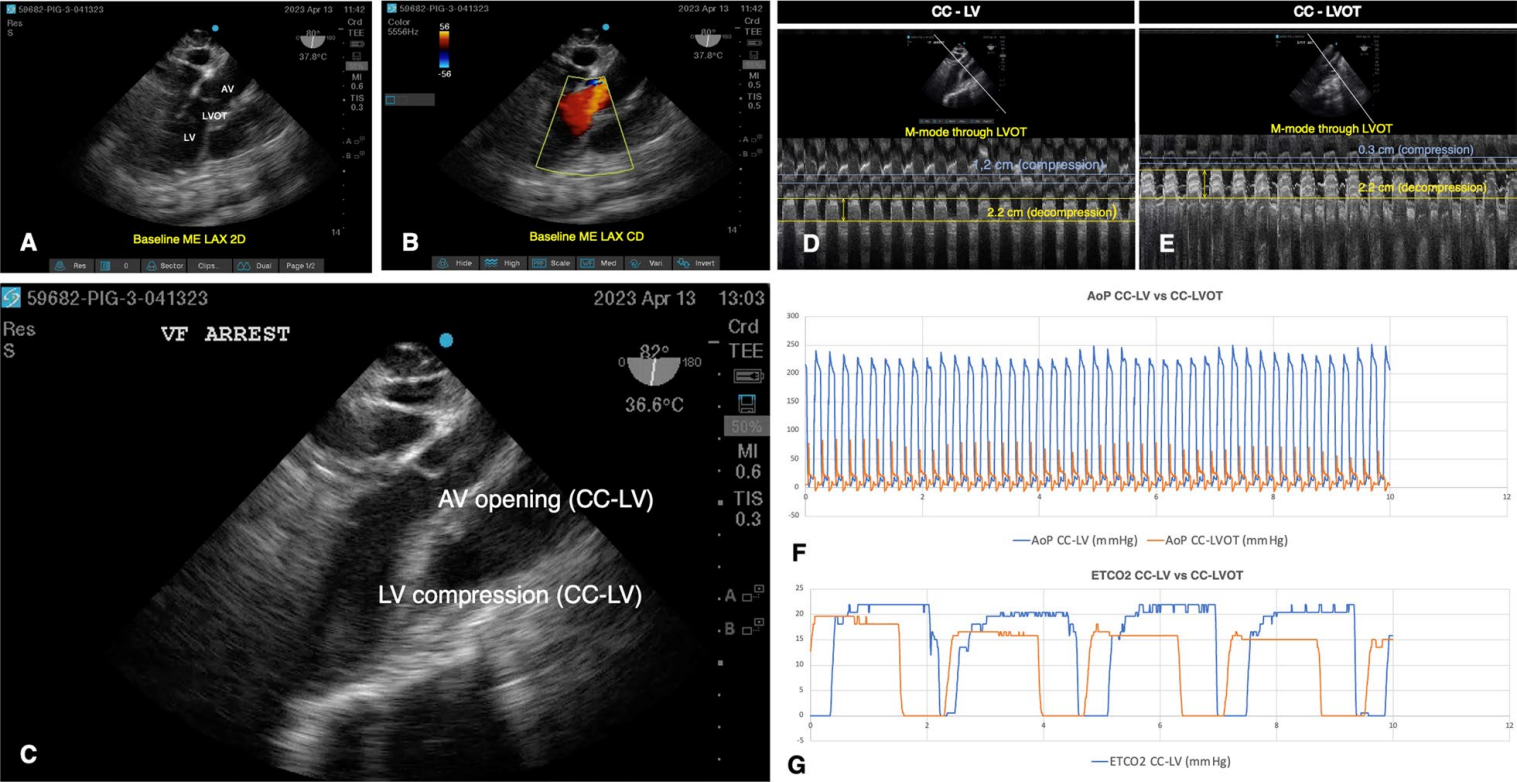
Summary of echocardiography and hemodynamic data. A TEE 2D image of midesophageal long axis view (MELAX) during baseline period depicting anatomic structures of interest: the left ventricle (LV), the left ventricular outflow tract (LVOT) and the aortic valve (AV). B MELAX view depicting Color Doppler (CD) through the LVOT. Red color in the Doppler scale corresponds to direction of blood flow from the LV, through the LVOT, and into the ascending aorta. C Shows MELAX image of mid-compression cycle when CCs were being directed to the LV (CC-LV). Anteroseptal wall of the LV is being compressed against the inferolateral wall of the LV and the AV is beginning to open. D and E M-mode image through the LVOT during CC-LV (D) and CC-LVOT (E) with unidimensional measurements of the diameter of this structure during the compression (yellow lines) and decompression (blue lines) phases of CPR. Overlapping hemodynamic measurements corresponding to simultaneous 10 s epochs comparing the two CC locations are shown in F (aortic pressures) and G (ETCO_2_)
